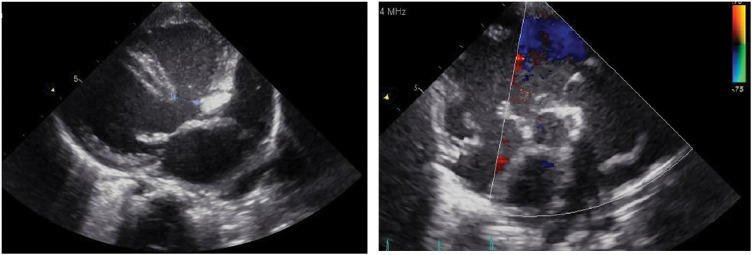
Preoperative transthoracic echocardiogram of patient 2. The echocardiogram shows a perimembranous VSD and dilated LV and RV. LV, left ventricle; RV, right ventricle; VSD, ventricular septal defect
